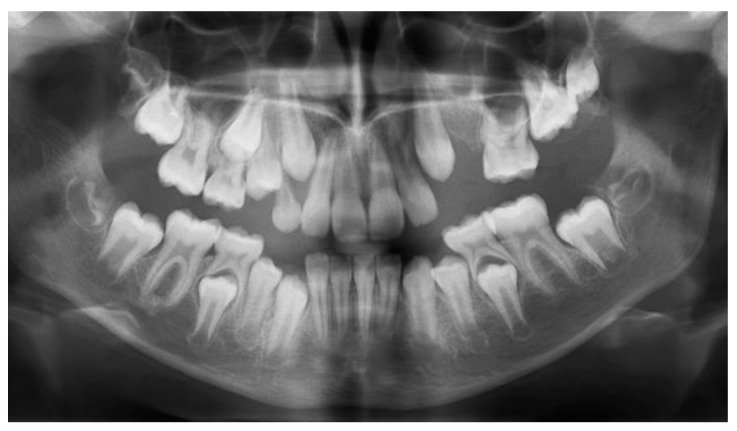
Panoramic X-ray at the age of 11 y and 8 m showed delayed development of 26 and 27.
